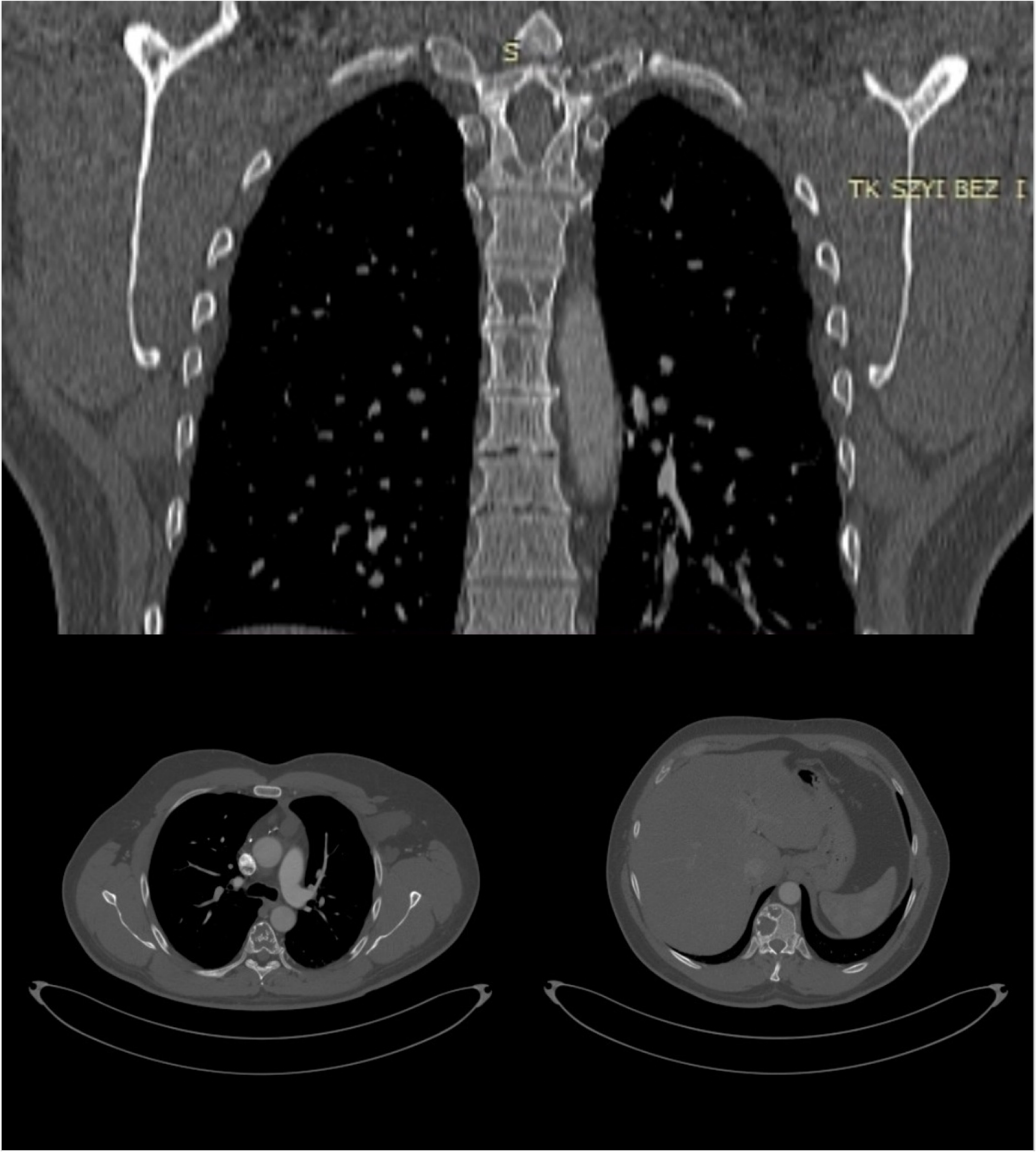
A CT scan of thoracic vertebrae displaying massive osteolytic lesions.
